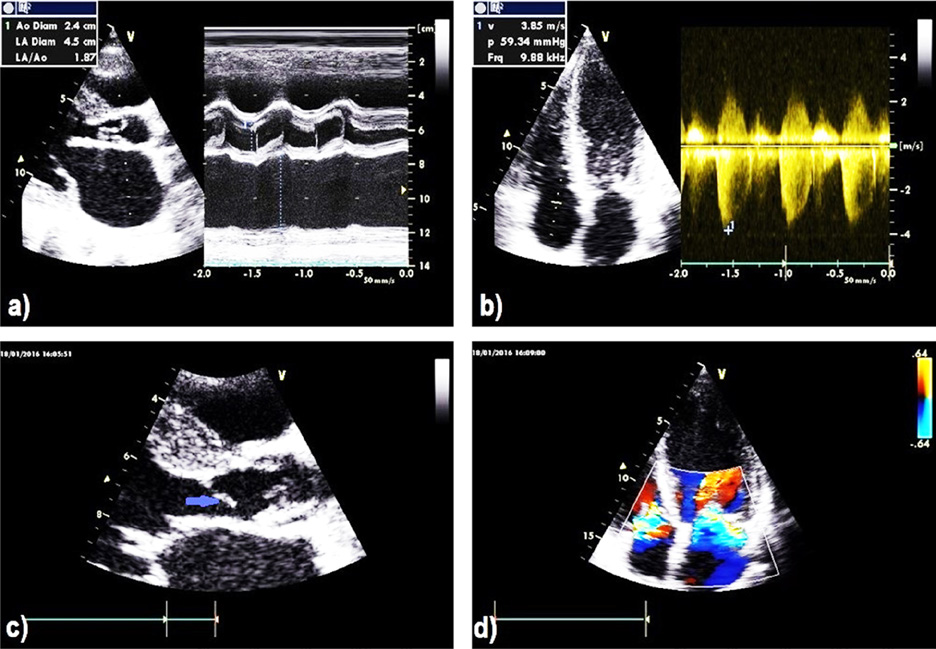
Transthoracic echocardiography (TTE) images show a) M-mode revealing dilated left atrium, b) Tricuspid regurgitation with severe pulmonary hypertension, c) multiple masses on the aortic valve, the longest of 20 mm implanted like a membrane (arrow), d) Color flow mapping (CFM) revealing severe tricuspid regurgitation (TR) and mitral regurgitation (MR).
